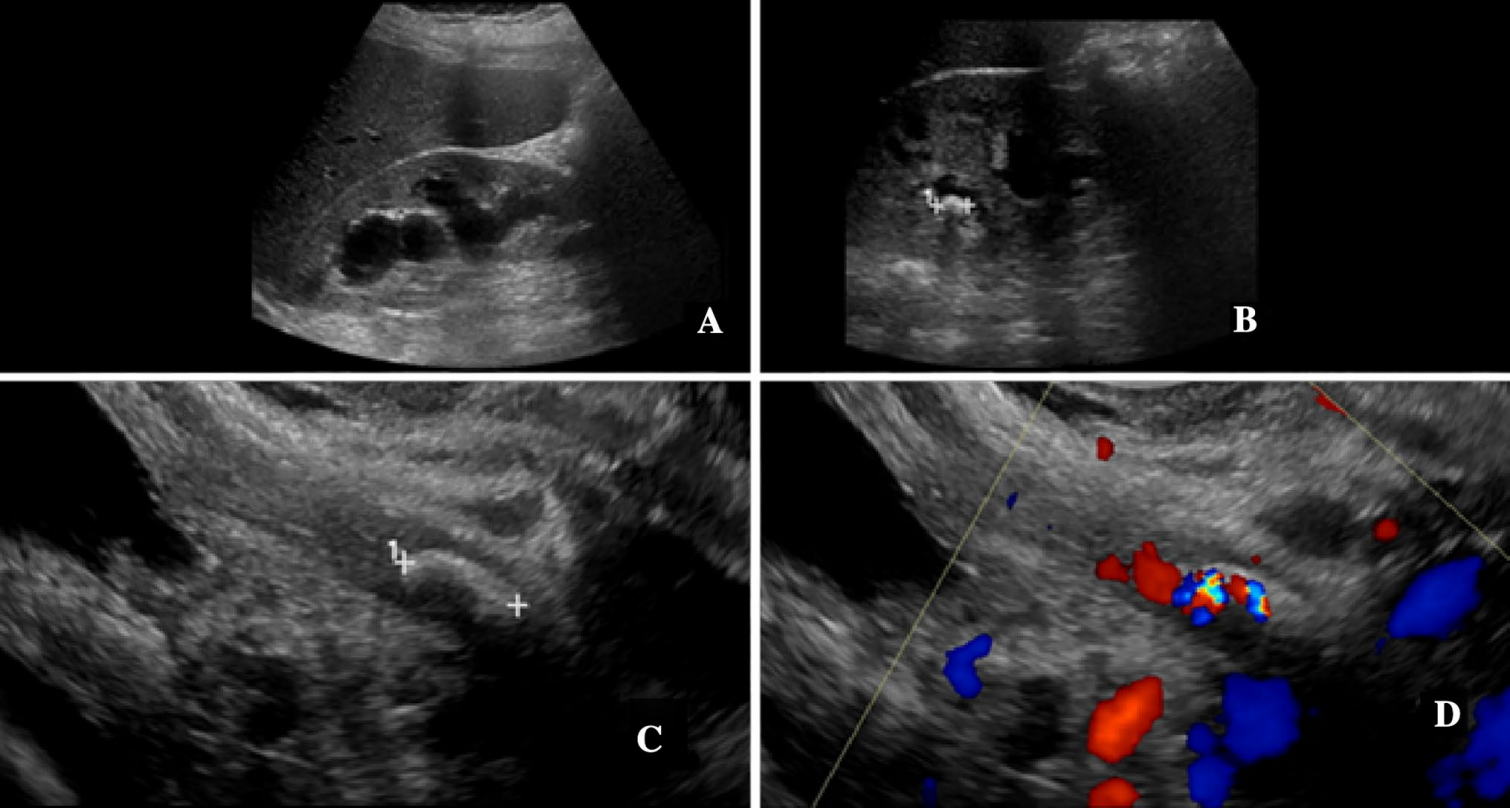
A 24-year-old G2P1001 presented at 30 weeks gestational age with right-sided renal colic. a Sagittal sonographic image of the right kidney demonstrated moderate hydronephrosis. b Additional sagittal image of right kidney shows an intrarenal stone, indicated by calipers. The transabdominal view of the bladder and ureterovesicular junction regions were reported negative. Subsequent sagittal transvaginal images in grayscale (c) and with color Doppler (d) demonstrate a stone (calipers) in the distal right ureter with twinkle artifact on color Doppler image. The patient was treated with cystoscopy, right ureteroscopy (which confirmed the stone), and stent placement. The patient carried the pregnancy to full term without complication
